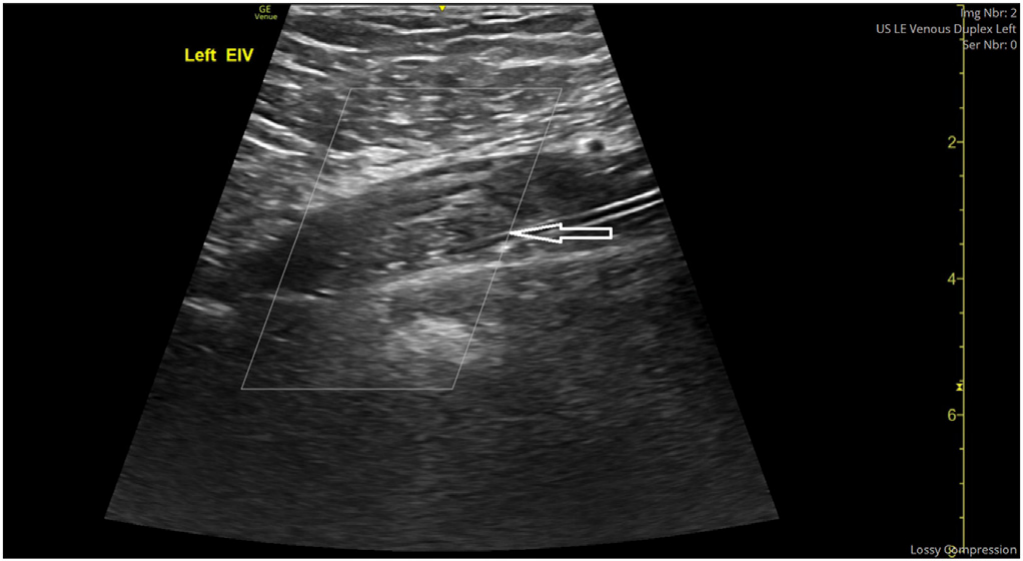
Left thigh ultrasound showing clot (white arrow) in the left external iliac vein. EIV, external iliac vein.
